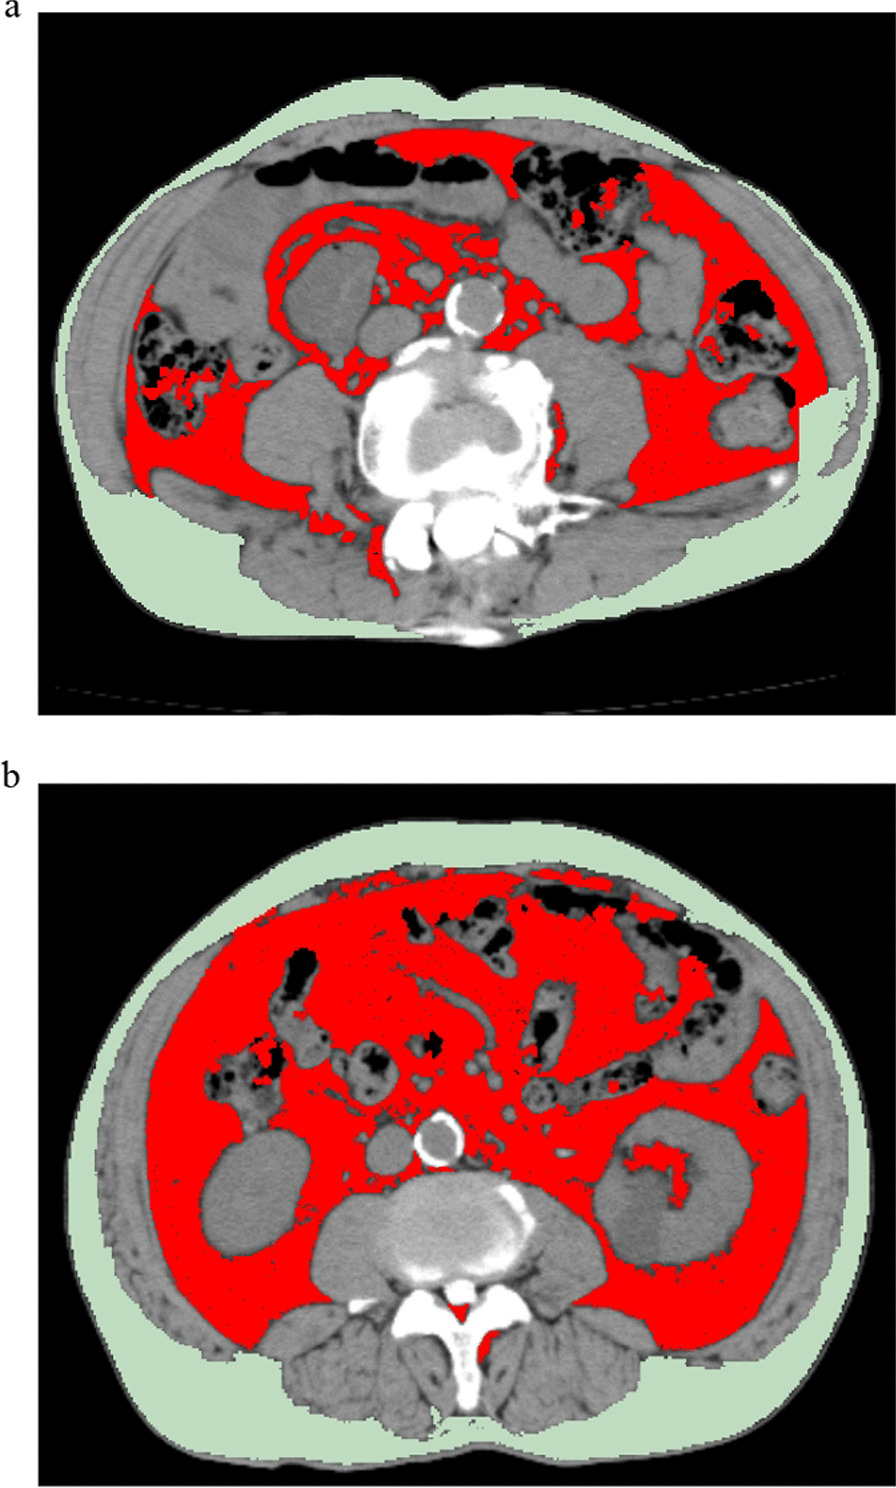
Abdominal CT images showing fat measurements (red: visceral fat; green: subcutaneous fat) in patients with low CSI scores (a) and high CSI scores (b). CT, computed tomography; CSI, central sensitization inventory
